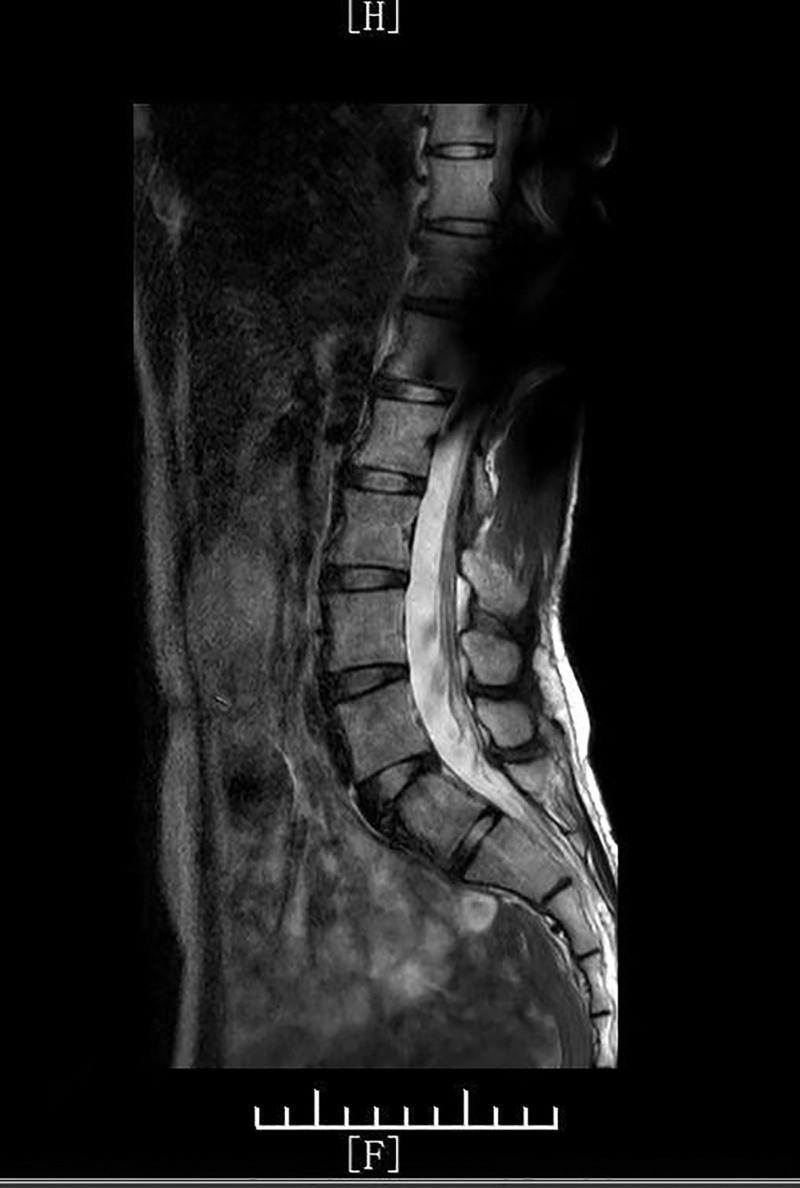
The density of the lumbar and sacral vertebral bodies is normal on the MRI, the L4–L5 intervertebral disc is slightly degenerated, the lumbar canal is not narrowed, and no other obvious abnormalities are seen. L4–5 = lumbar 4 to 5, MRI = magnetic resonance imaging.
